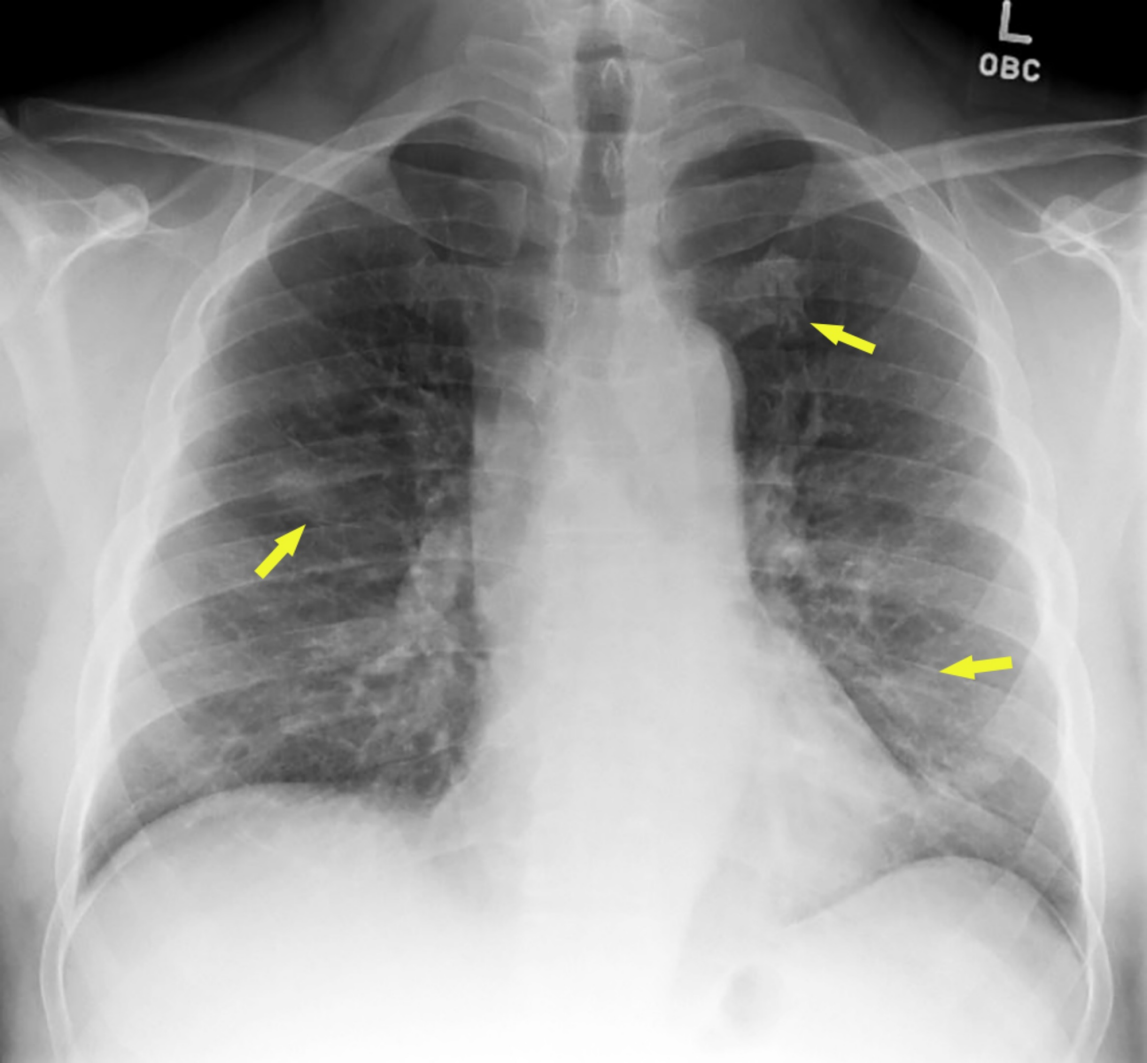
Chest X-ray showing hazy bilateral lung opacities (arrows)
